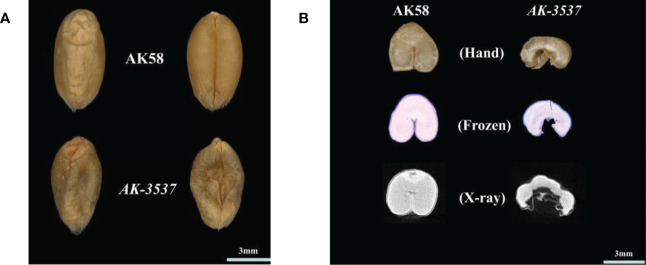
Wheat grain Dek phenotype and the internal structure of AK58 and *AK-3537*. (A) Dek phenotype of *AK-3537* mature grain compared to AK58 normal grain. (B) The internal structure of AK58 and *AK-3537* mature grains was observed by free-hand section (Hand), frozen section (Frozen), and x-ray tomography (X-ray).
